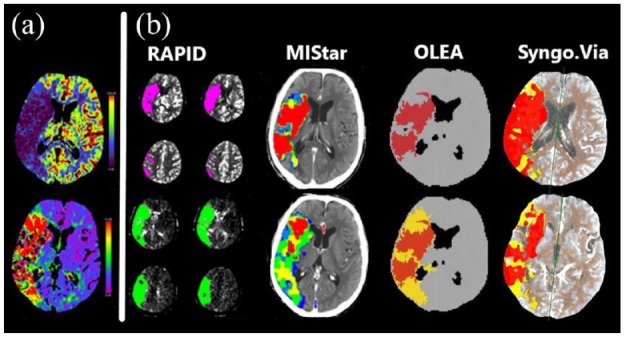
Computed tomography perfusion imaging in a 72-year-old male with occlusion of the right internal carotid artery and proximal M1-branch of the middle cerebral artery (tandem occlusion): (a) cerebral blood flow (upper row) and time-to-maximum (lower row) and (b) ischemic core volumes (upper row, red or magenta) and perfusion lesion volumes (lower row, green and yellow) by different automated imaging analysis software packages.
